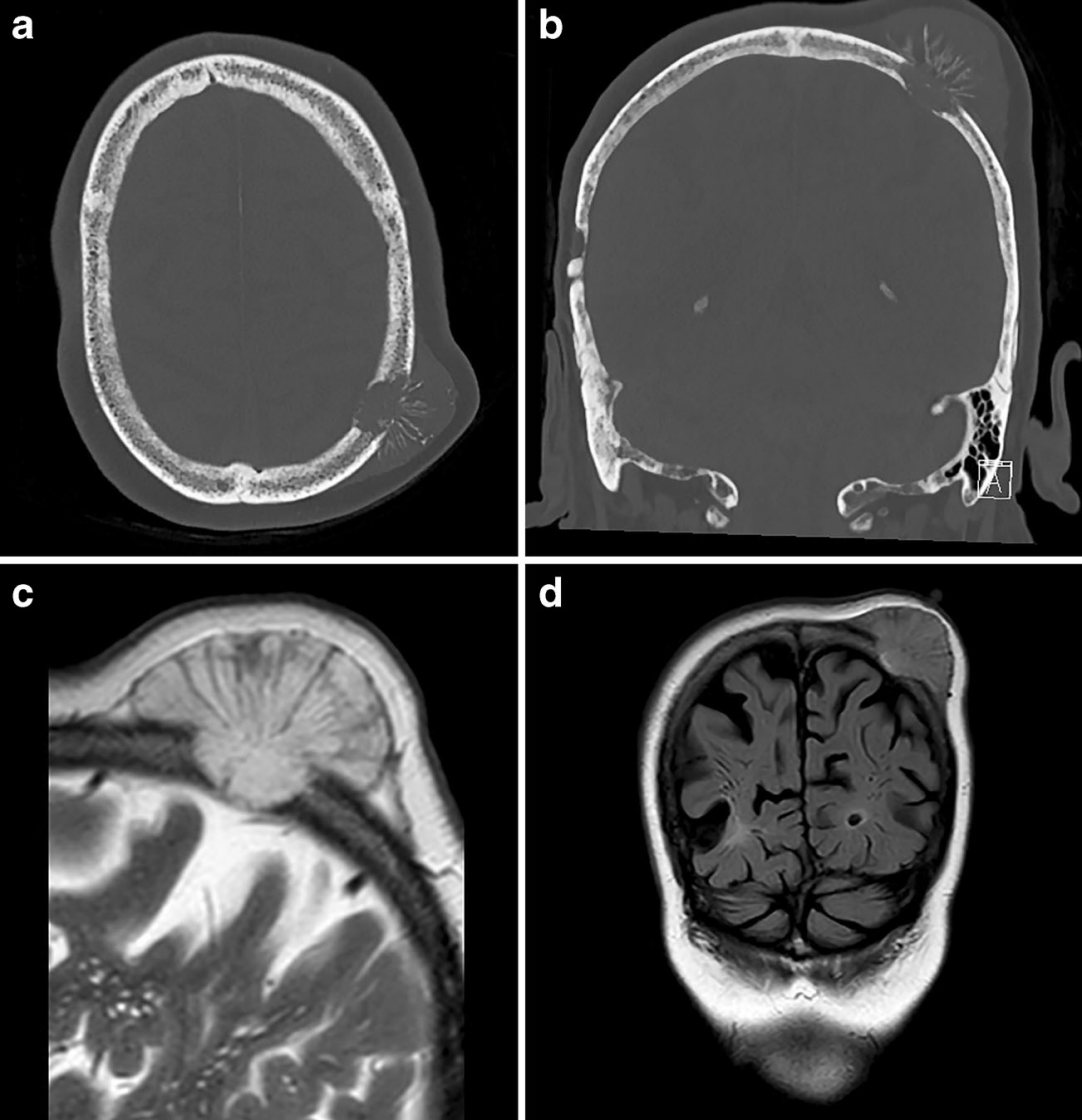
a Transverse and b coronal CT, windowed to bone setting, demonstrating destruction of both the external and internal tabula of the skull. Extracranial radial striped calcified fragments are present. c Sagittal T2-weighted and d coronal FLAIR MRI, demonstrating growth to the level of the dura mater, without parenchymal involvement
